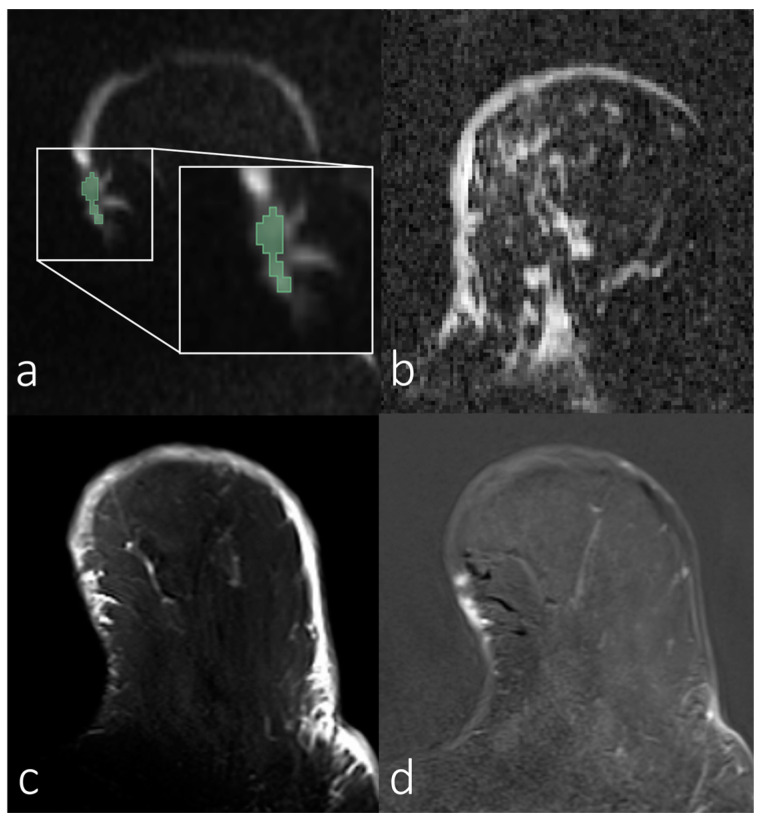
Breast magnetic resonance images of a woman with skin infiltrating mammary carcinoma. (a) Manual segmentation overlaid on the diffusion-weighted image (b = 800 s/mm^2^), with segmentation highlighted in green; (b) apparent diffusion coefficient map; (c) T_2_-weighted image with fat saturation; (d) T_1_-weighted subtraction image after contrast administration.
